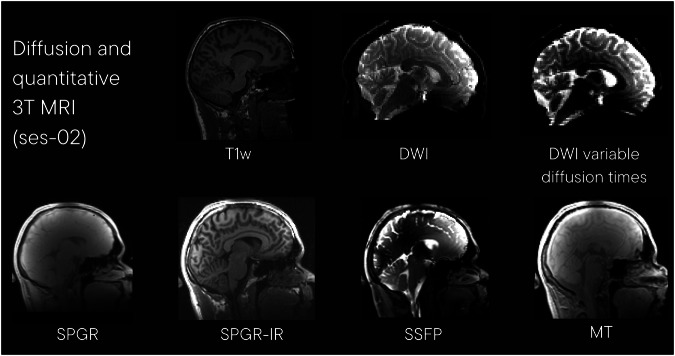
Example images from session 02, Diffusion and quantitative MRI using ultra strong magnetic gradients. T1w: T1-weighted structural image (acquired with MPRAGE); DWI: diffusion weighted image; SPGR: 3D spoiled gradient-recalled echo sequence; SPGR-IR: inversion recovery-prepped spoiled gradient-recalled echo sequence; SSFP: steady-state free precession; MT: quantitative magnetization transfer image.
